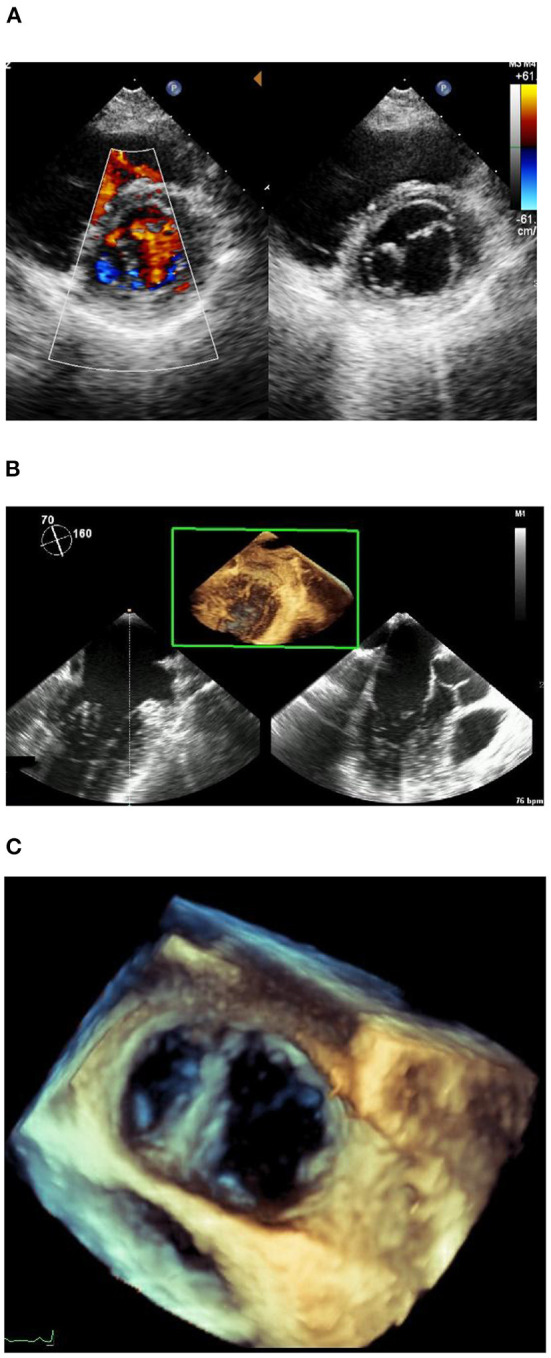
(A) Short axis of echocardiogram image. (B) Transesophageal echocardiogram. (C) Transesophageal echocardiogram.
